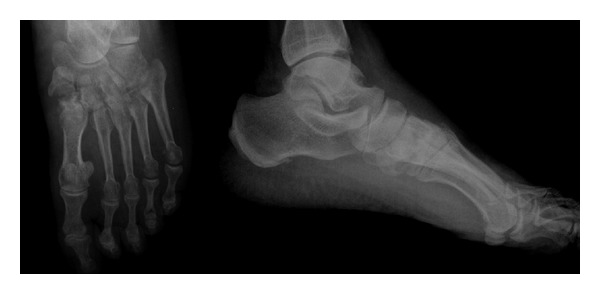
Preoperative anteroposterior and lateral X-ray.
